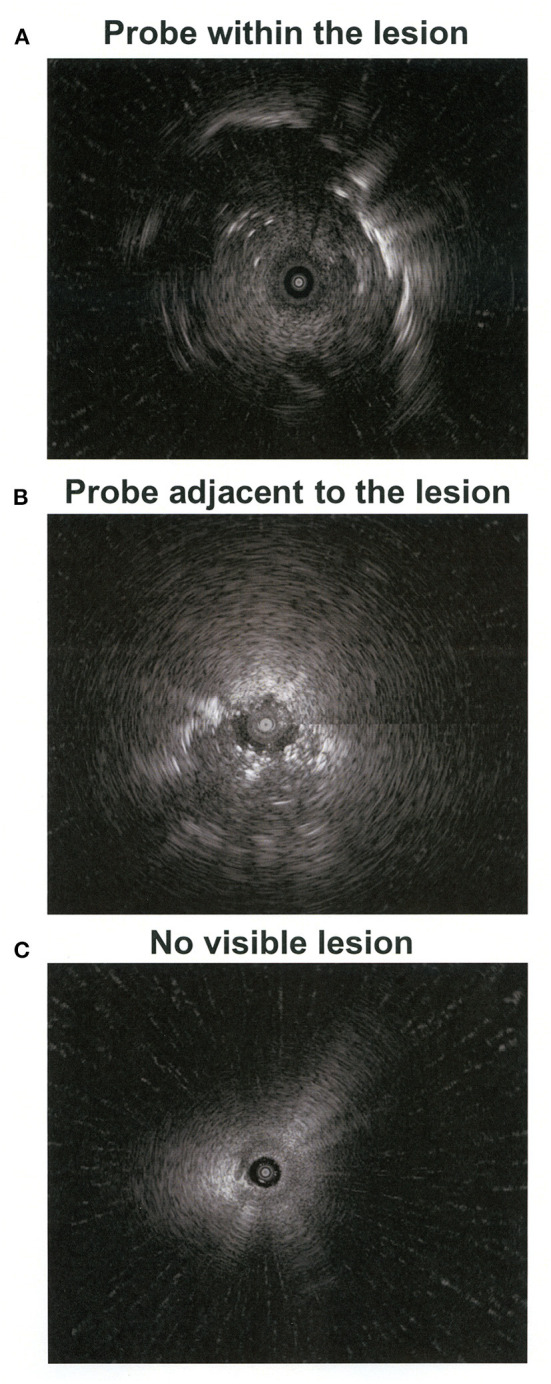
Images of the radial-probe endobronchial ultrasound. The central black cycles (A–C) indicate the probe position and the hyperechoic areas (A,B) show the lesion.
